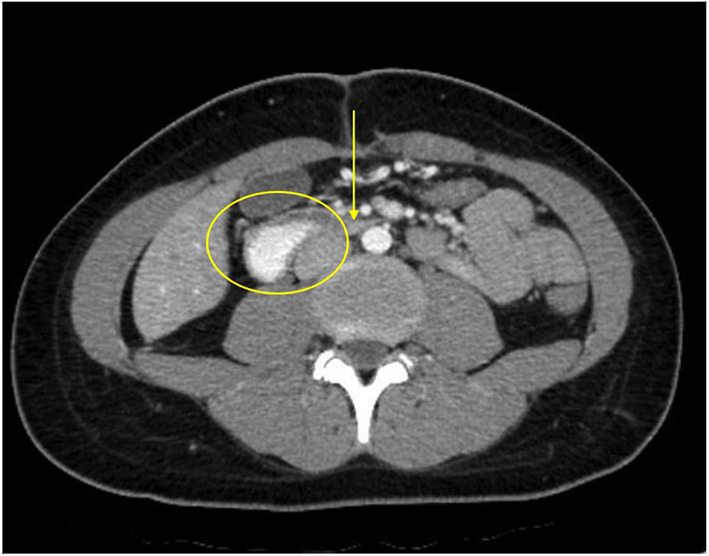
The patient's axial CT scan with oral contrast demonstrates the classic findings of superior mesenteric artery syndrome. The arrow points to the narrowed third segment of the duodenum, compressed between the superior mesenteric artery anteriorly and the abdominal aorta posteriorly. The duodenum proximal to the compressed segment is dilated and filled with contrast (circle).
